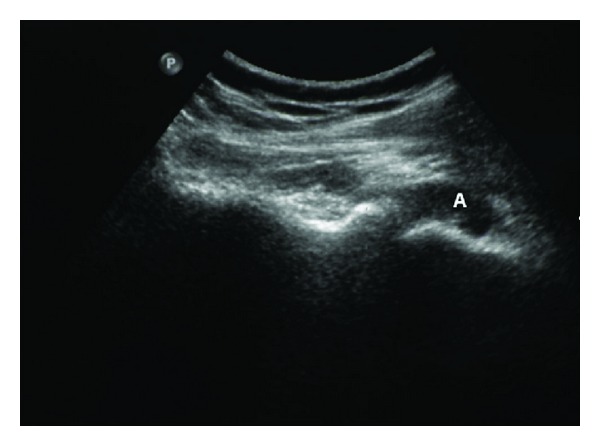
Ultrasound of the right shoulder joint using an axial posterior approach to the glenohumeral articulation showing a markedly distended posterior aspect of joint capsule by fluid (A).
